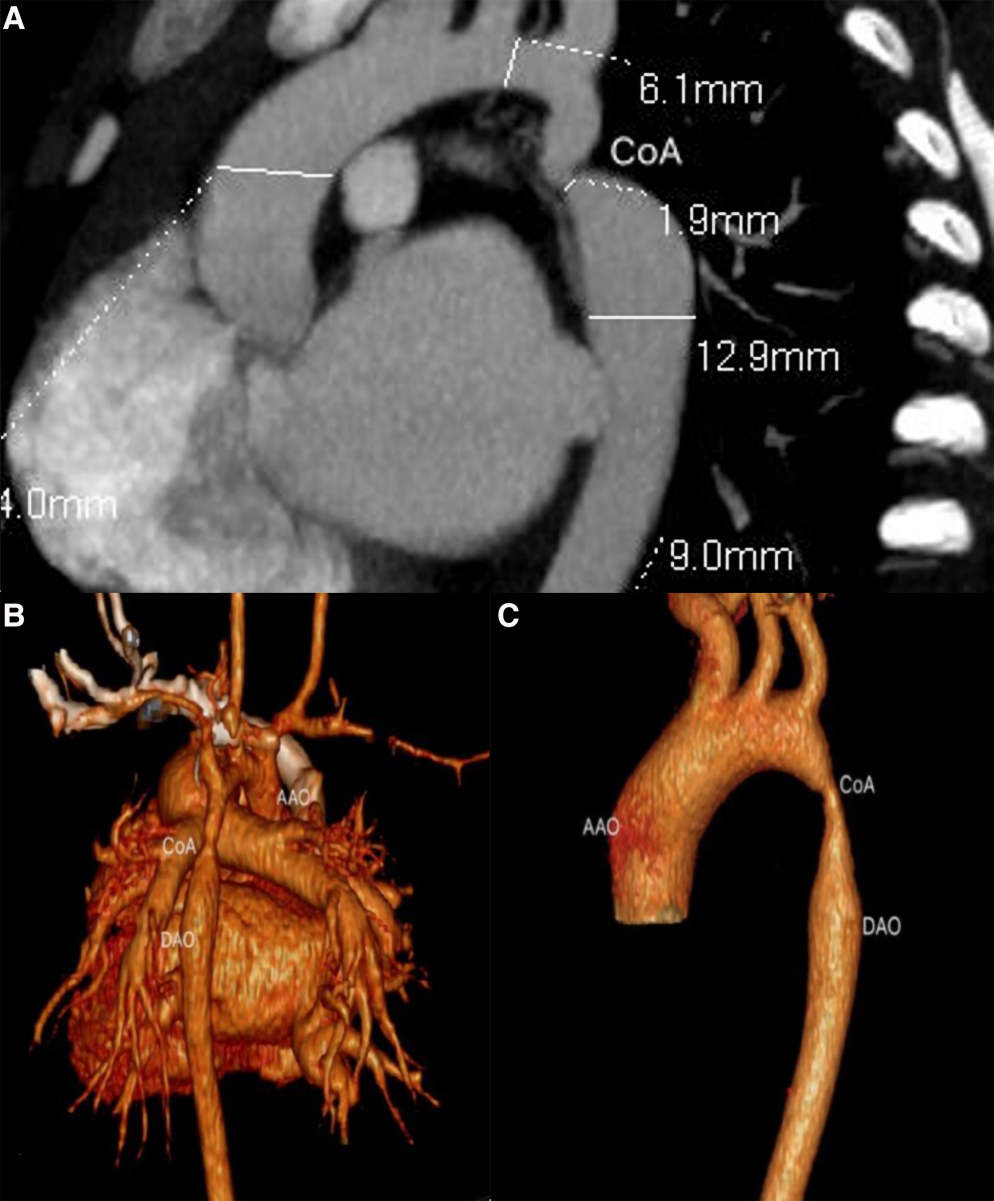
Ductal CoA by CTA. (A) The anatomical measurements by CTA before cardiac surgery; (B,C) sagittal multiplanar reconstruction showed volume rendering images with CoA.
